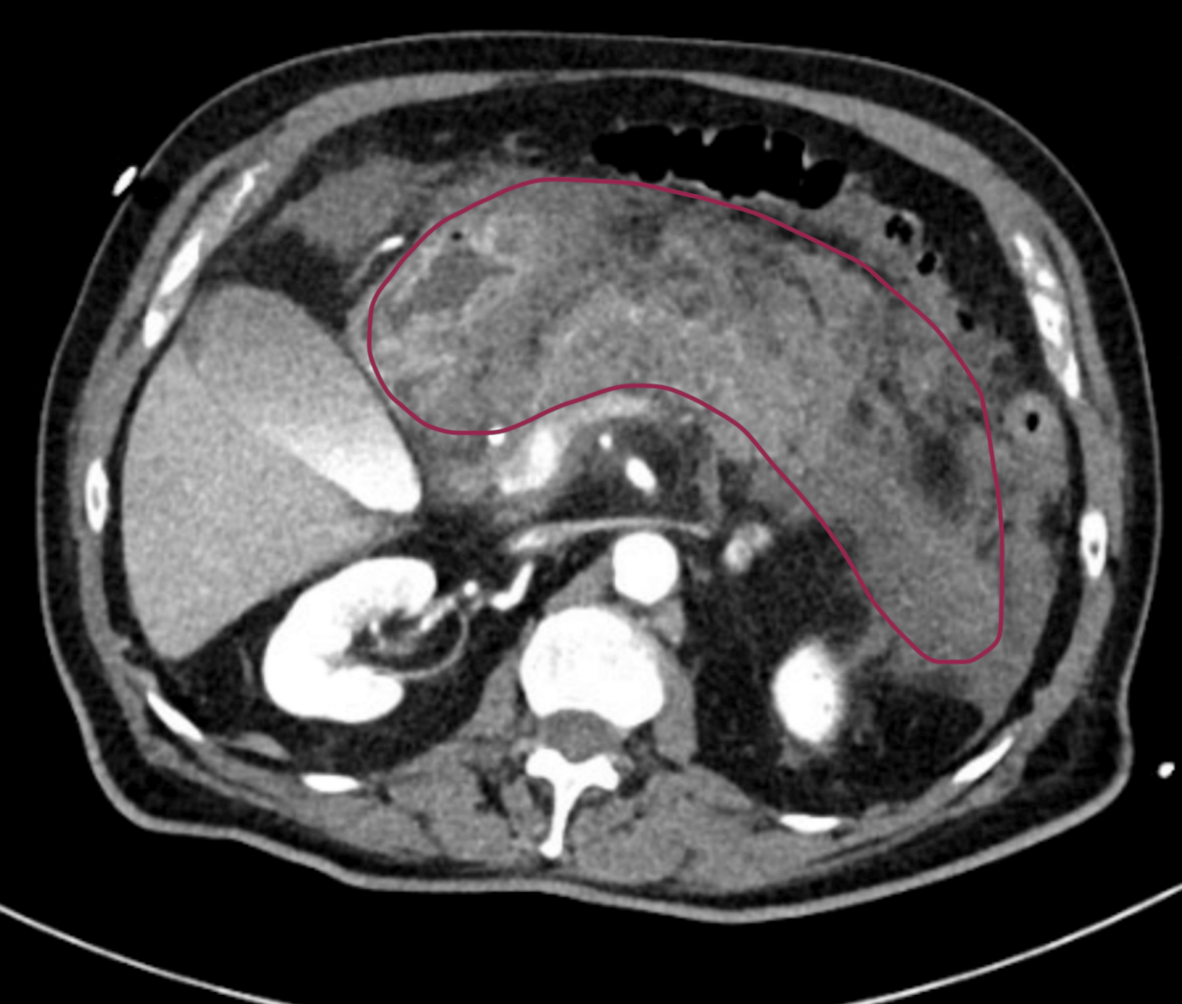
Abdominal CT scan showing extensive necrohemorrhagic pancreatitis Abdominal CT scan showing necrohemorrhagic pancreatitis, as shown by lined delimitation, requested after clinical worsening of the patient.
